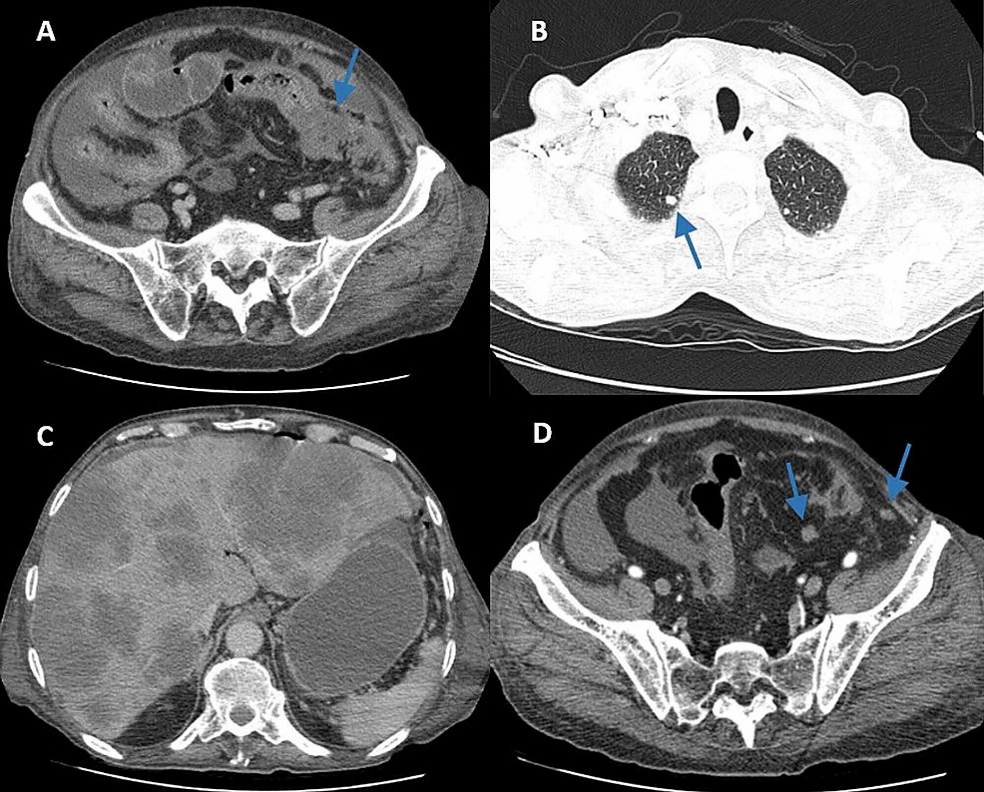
CT-scan images CT-scan images compatible with sigmoid neoplasia (A); pulmonary metastasis (B); liver metastasis (C); and peritoneal carcinomatosis (D)
